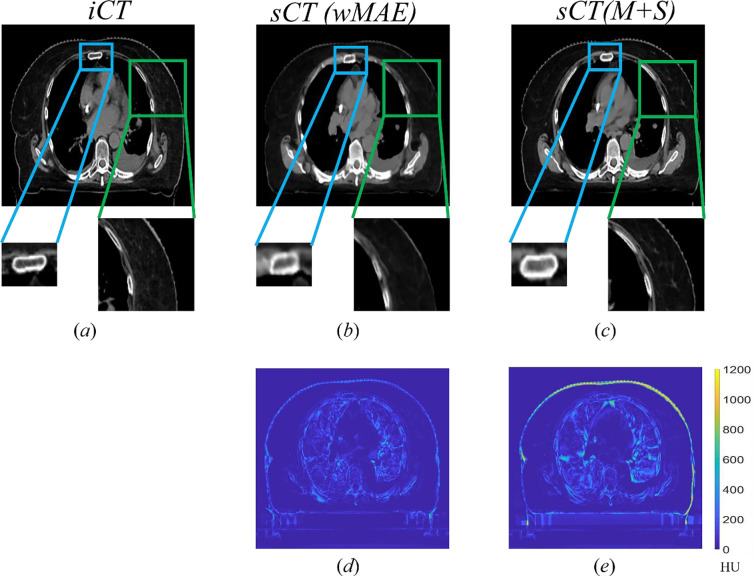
Comparison of a typical slice between the iCT slice (*a*) and its corresponding sCT generated by the model trained with wMAE only (wMAE) (*b*) and the model trained with both wMAE and SSIM (M+S) (*c*), respectively. The CT HU number display window level and width were −125HU and 1300HU respectively. The edge of the rectum was more blurred in sCT generated by the wMAE model. The high Z material in sCT generated by the M+S model were partially recovered, whereas the wMAE model did not. Fig. 5(d) and (e) showed the absolute difference of the slice between the iCT and sCT generated by the wMAE and (M+S) models, respectively.
